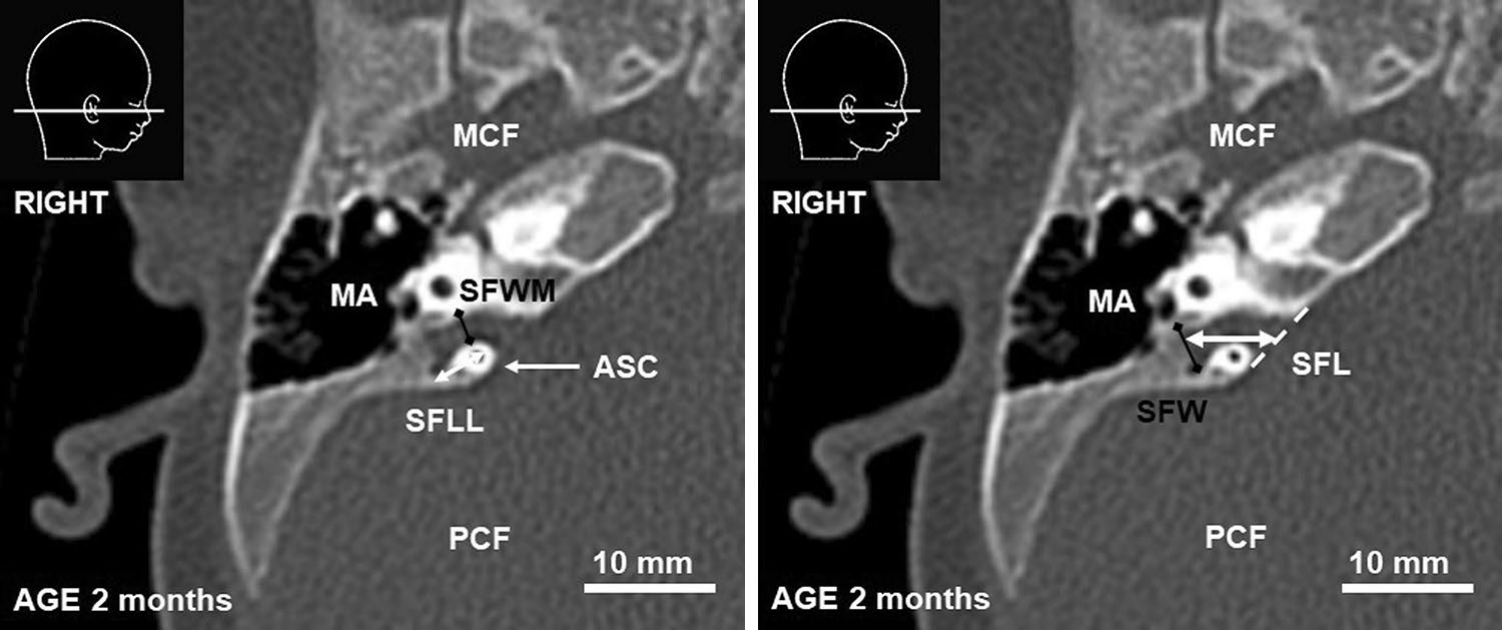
CT scan of a 2-month-old infant. *ASC* anterior semicircular canal, *MCF* middle cranial fossa, *MA* mastoid antrum, *PCF* posterior cranial fossa. SFLL, SFWM, SFW, SFL—parameters described in the text and Table 1
